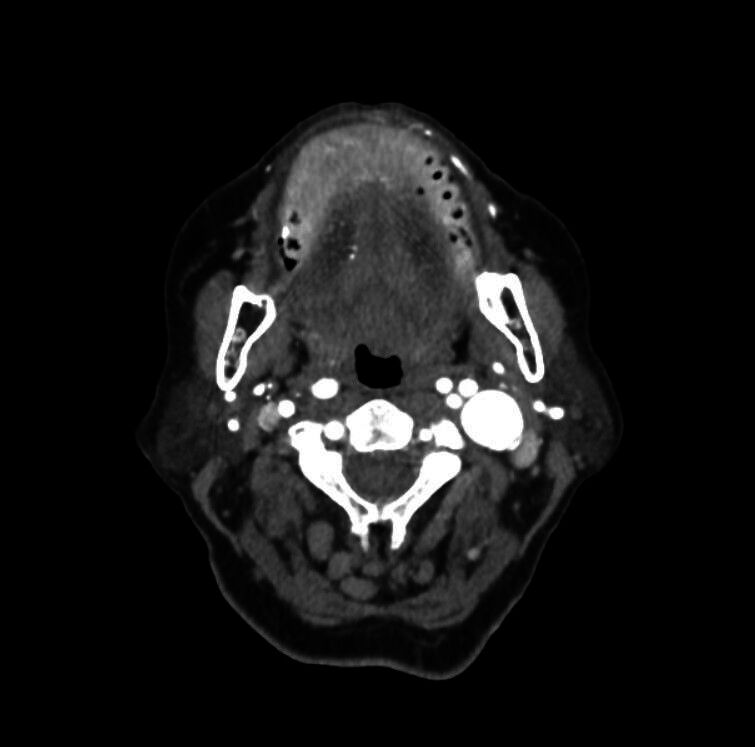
CTA showing a 20 × 20 mm tortuous aneurysm in the mid-left cervical ICA, extending towards the skull base. CTA: computed tomography angiography; ICA: internal carotid artery
